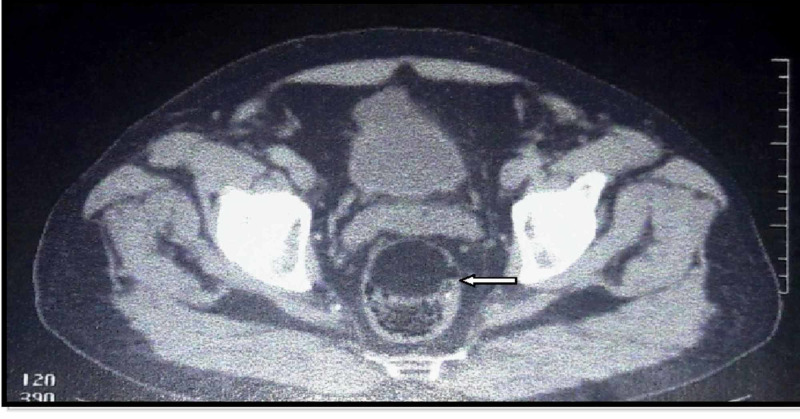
Contrast-enhanced abdominal CT showing a well-defined mass of 4.5 cm × 4.2 cm (arrow)
